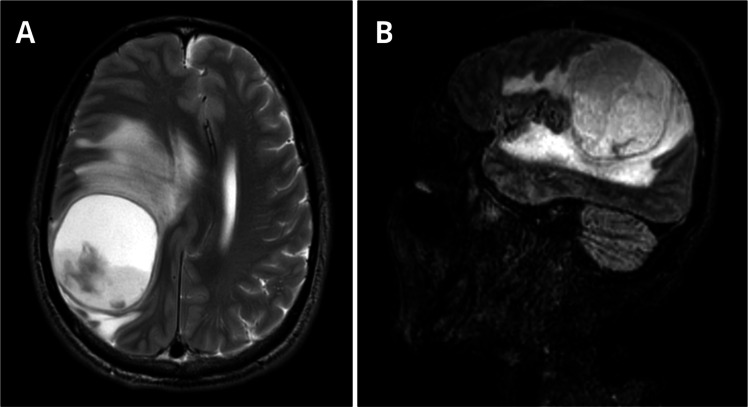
Axial T2-weighted MRI (A) shows a lesion measuring 5.9 × 5.4 × 5.4 cm (CC × LL × AP) and exerts a compressive effect on the brain parenchyma, with hyperintense areas in the white matter secondary to vasogenic edema, accompanied by subfalcine herniation and right uncal herniation into the suprasellar cistern. There is an approximate 1.7 cm midline shift to the left. Peripheral rim enhancement without surrounding capsule irregularity is observed, findings that are suggestive of a tuberculous abscess rather than a pyogenic one. Sagittal MRI on T1 complements the visualization of the lesion (B).
